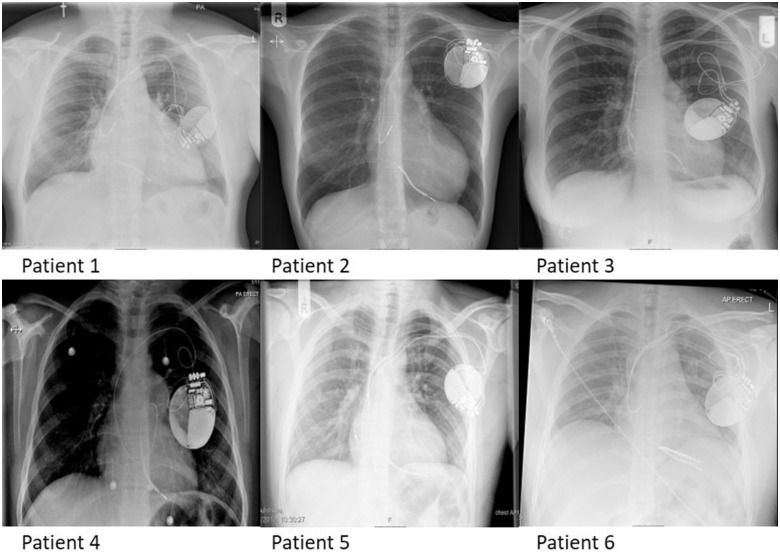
Anteroposterior chest radiographs performed for each patient.
